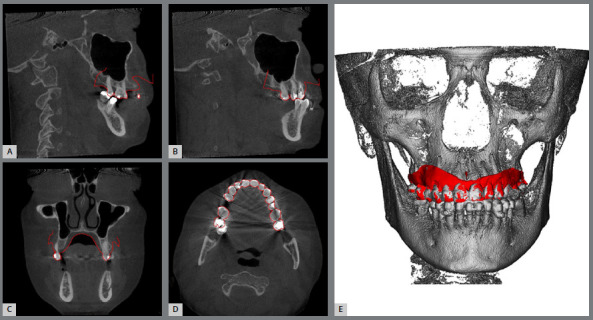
Superimposition of the maxillary STL model file on the CBCT images ( the red line is the external contour of the model ): A) right sagittal view; B) left sagittal view; C) coronal view; D) axial view; E) three-dimensional reconstruction, with the final position of the superimposed maxillary STL model.
